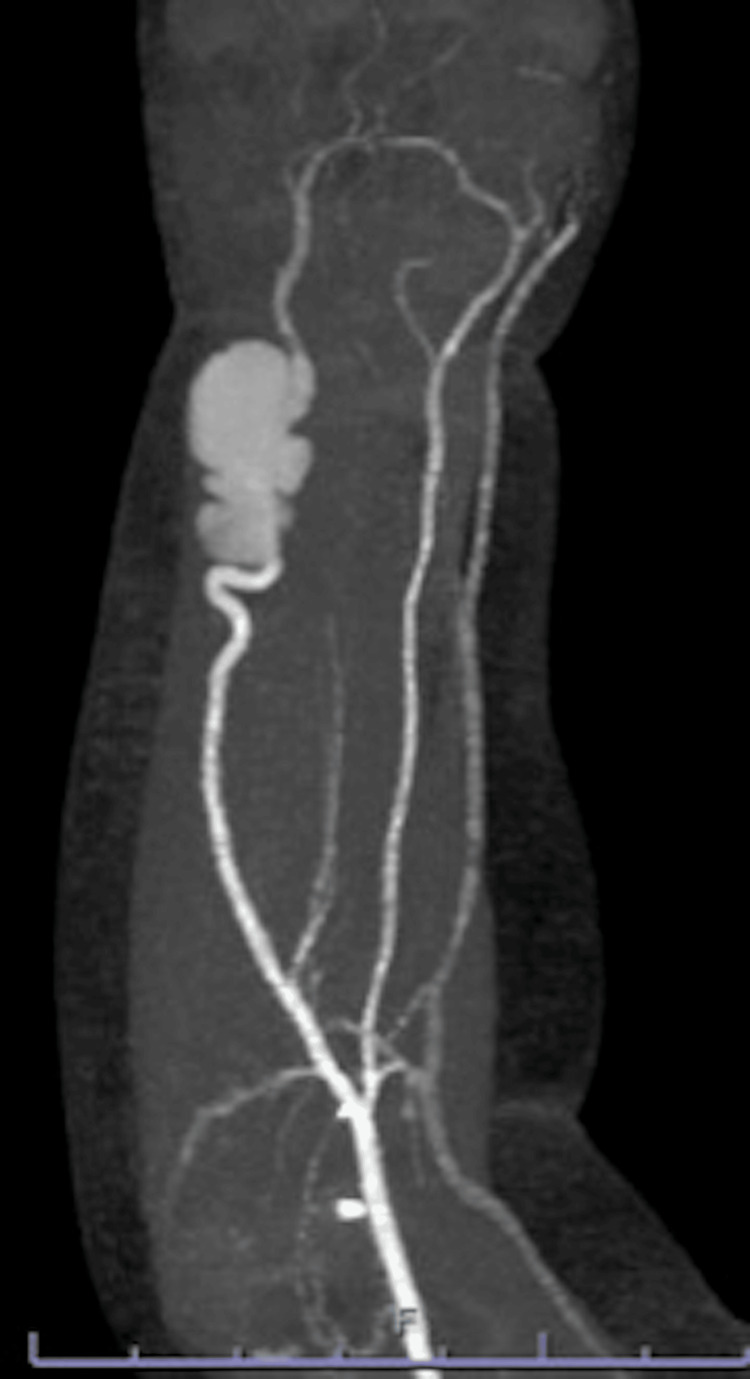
Preoperative contrast-enhanced CT of the right forearm. An ulnar artery aneurysm is evident. There is no sign of radial artery hypoplasia or other vascular anomaly.
